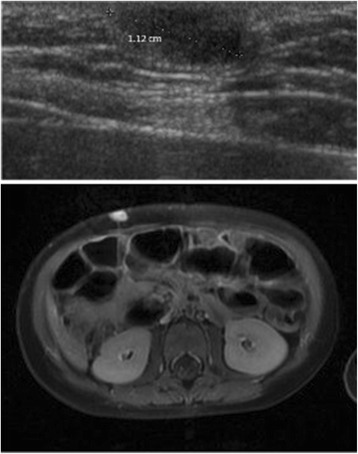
Ultrasound of the abdominal wall showing a subcutaneous oval-shaped nodule, hyporeflective, and without clear margins. Abdominal magnetic resonance imaging (axial oblique T1-weighted with contrast) with a contrast-captivating nodule in the right abdominal wall
